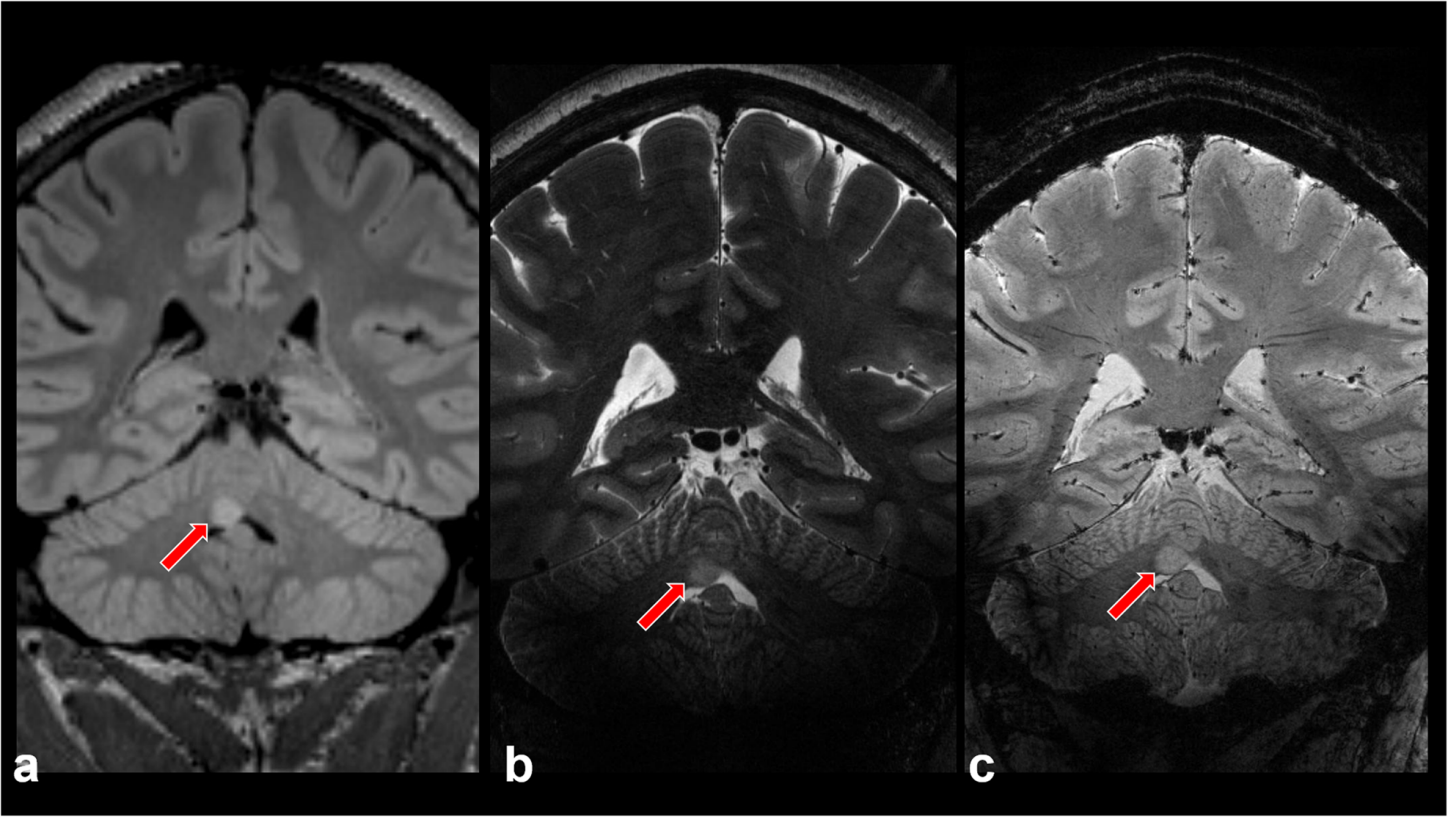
Hamartoma identified on 3-T magnetic resonance imaging and further characterized at 7 T. Coronal T2-weighted fluid-attenuated inversion-recovery image at 3 T (a) demonstrates a hyperintense mass (red arrows) in the right cerebellar vermis. Coronal T2-weighted (b) and coronal susceptibility-weighted image (c) obtained at 7T show no evidence of microvascularity within the lesion
